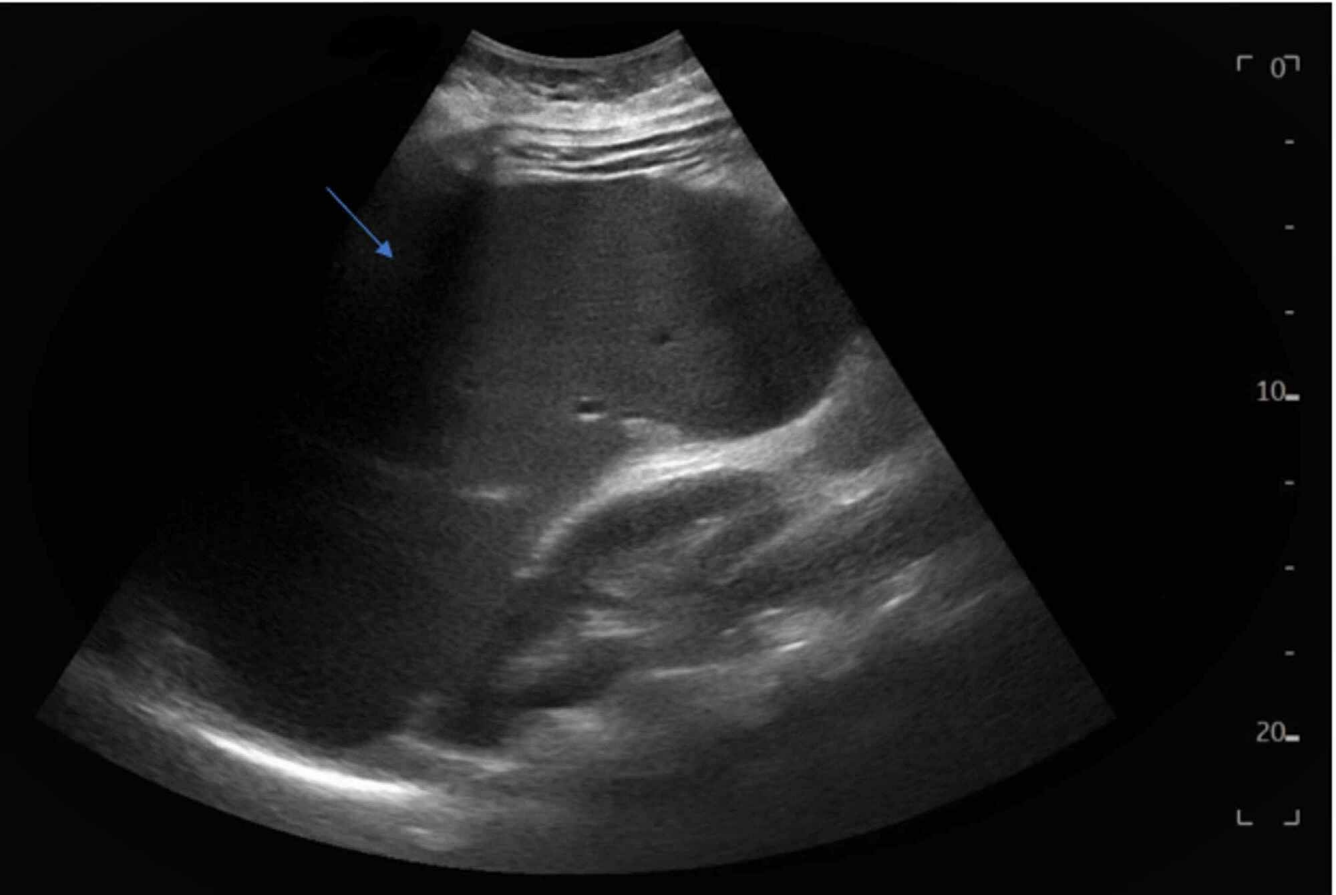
Abdominal ultrasound showing splenomegaly (arrowhead)
